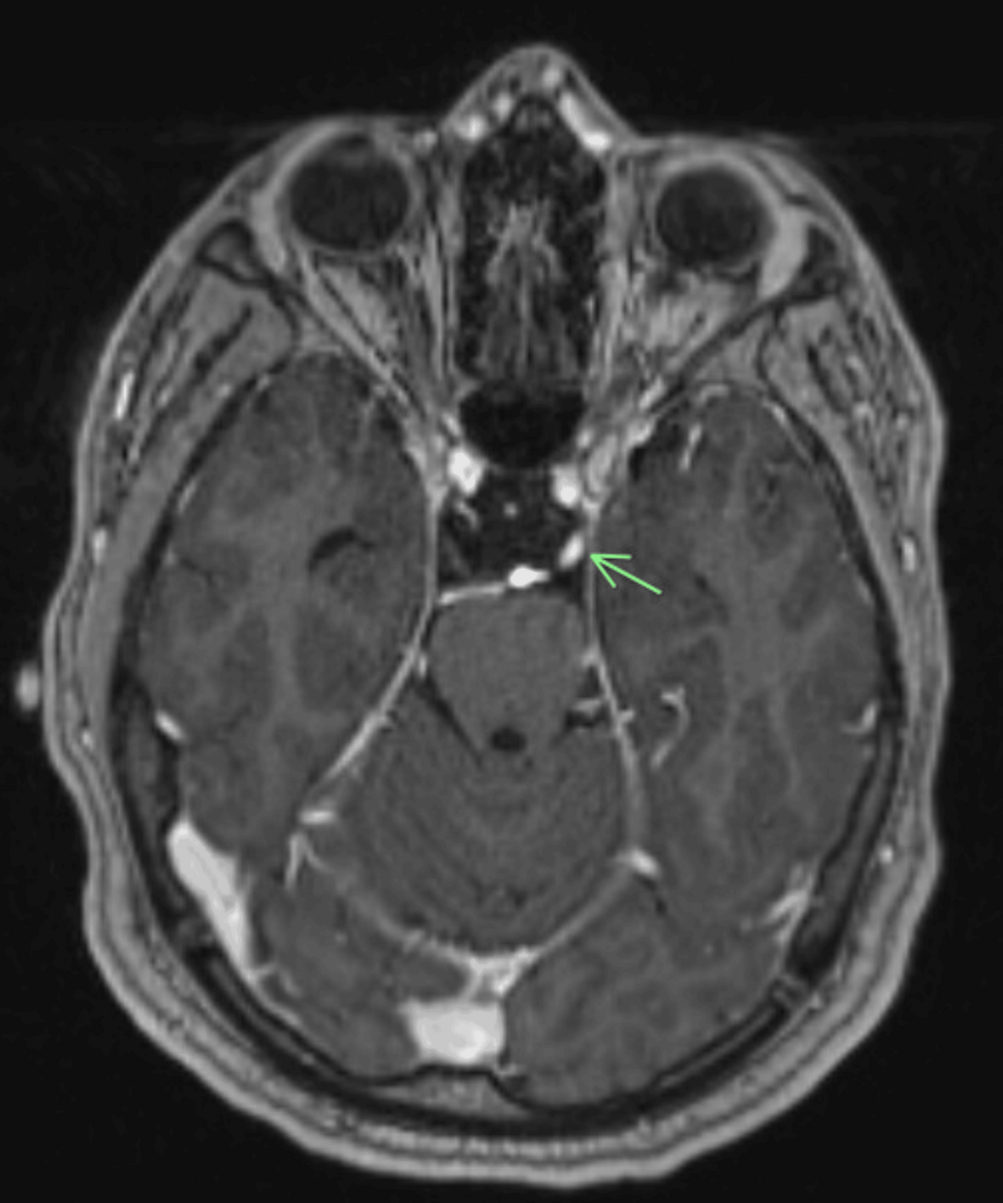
Brain magnetic resonance imaging (MRI) showing abnormal enhancement along the left oculomotor nerve (green arrow), raising concern for leptomeningeal disease.
